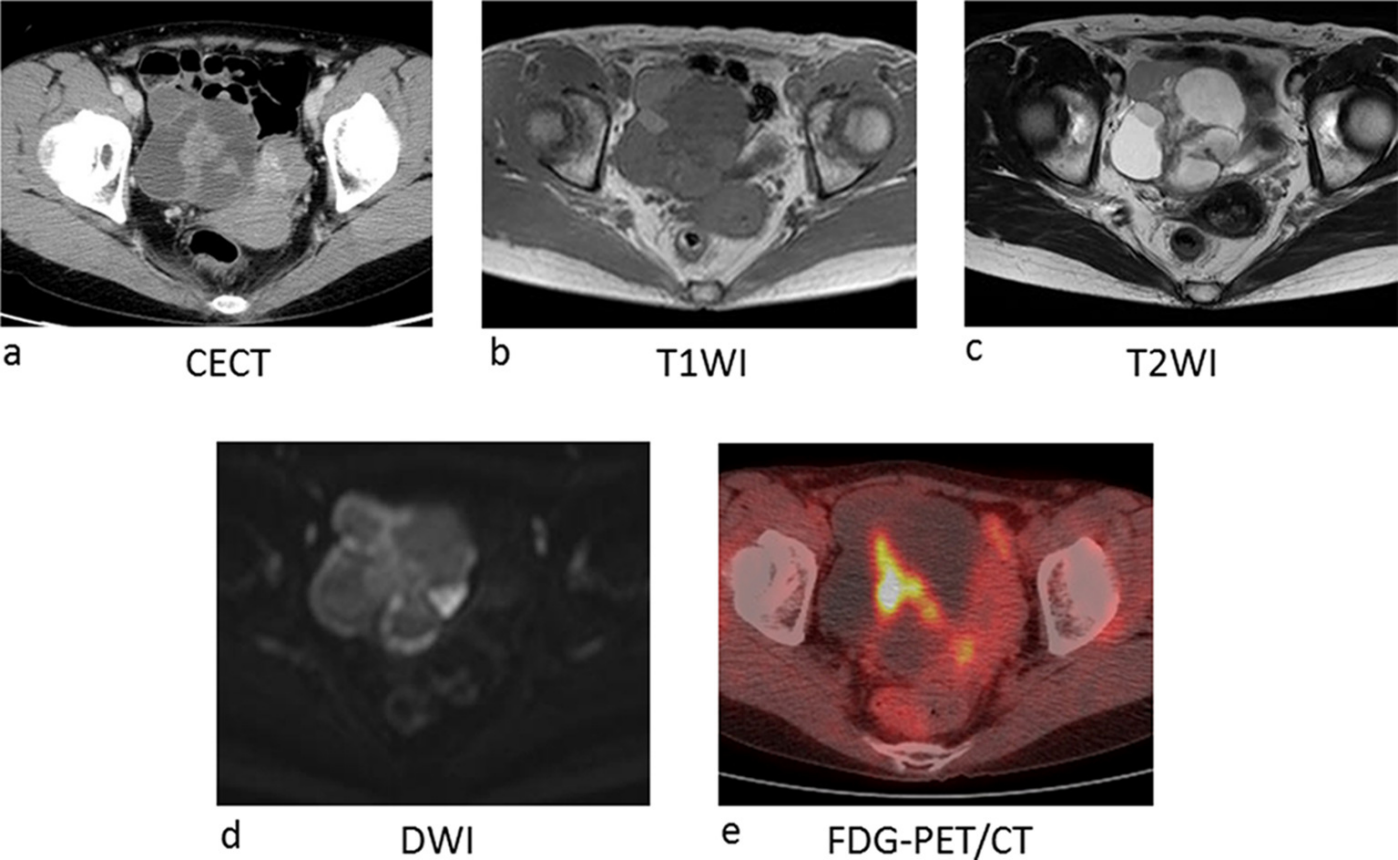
Imaging of Case 3. (a) On contrast enhanced CT image, complex solid and cystic tumour is located at the right side of the uterus. On both *T*_1_ (b) and *T*_2_ weighted image (c), each locule shows different signal intensities with shading due to haemorrhage within the cyst. Papillary lesion was observed on the tumour septum on *T*_2_WI and enhanced on post contrast CT and higher signal intensity on Diffusion weighted image (d). (e) PET-CT shows strong uptake to the solid component. PET-CT, positron emission tomography CT.
